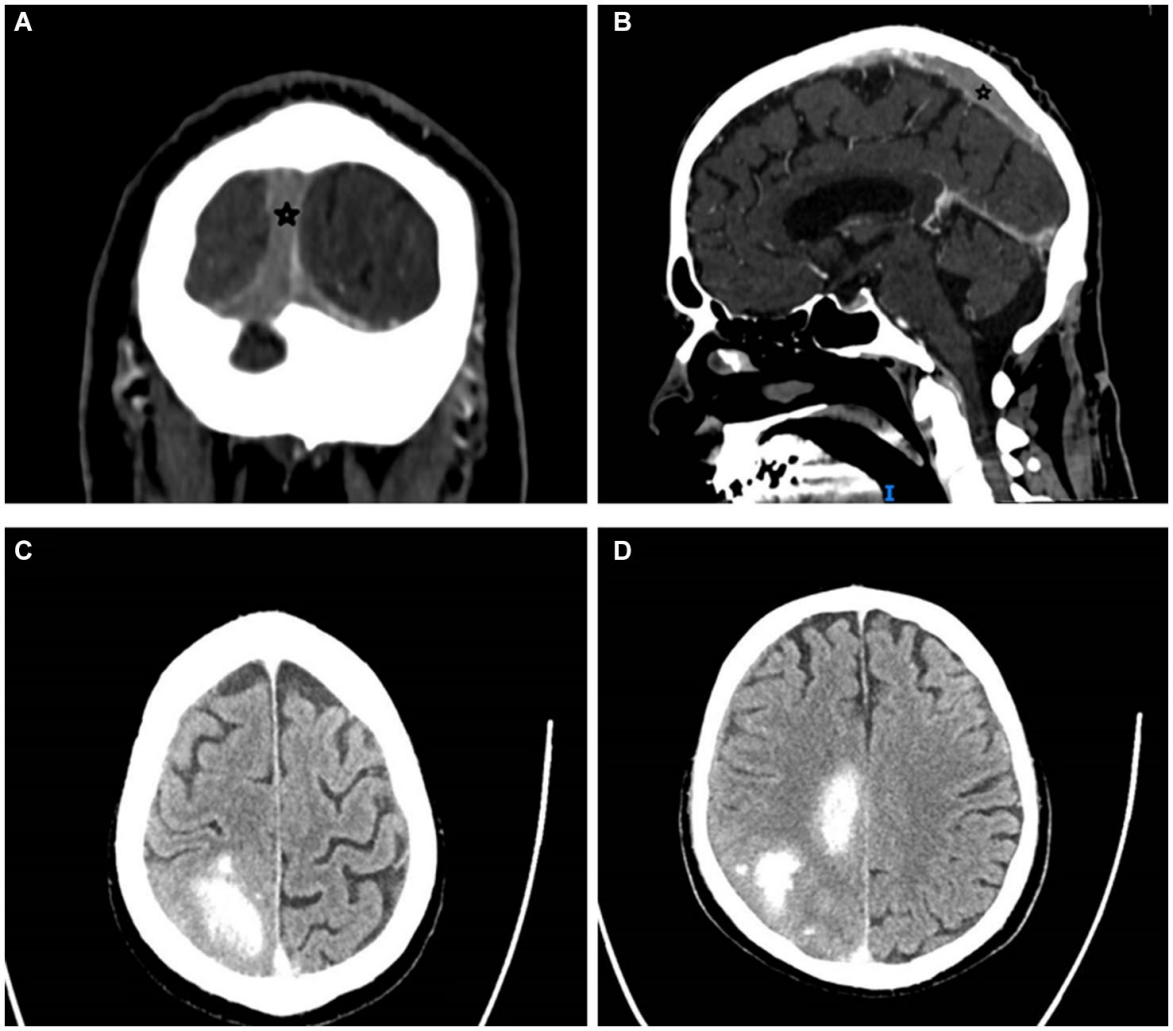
Cerebral sinus vein thrombosis at presentation. Star mark signifies sinus vein thrombosis. (A) Occluded sagittal sinus, coronal view, CT venography. (B) Occluded superior sagittal sinus, sagittal view, CT venography. (C,D) Right parenchymal hemorrhages and subarachnoid hemorrhage, transverse view, CT without contrast. CT, computed tomography.
